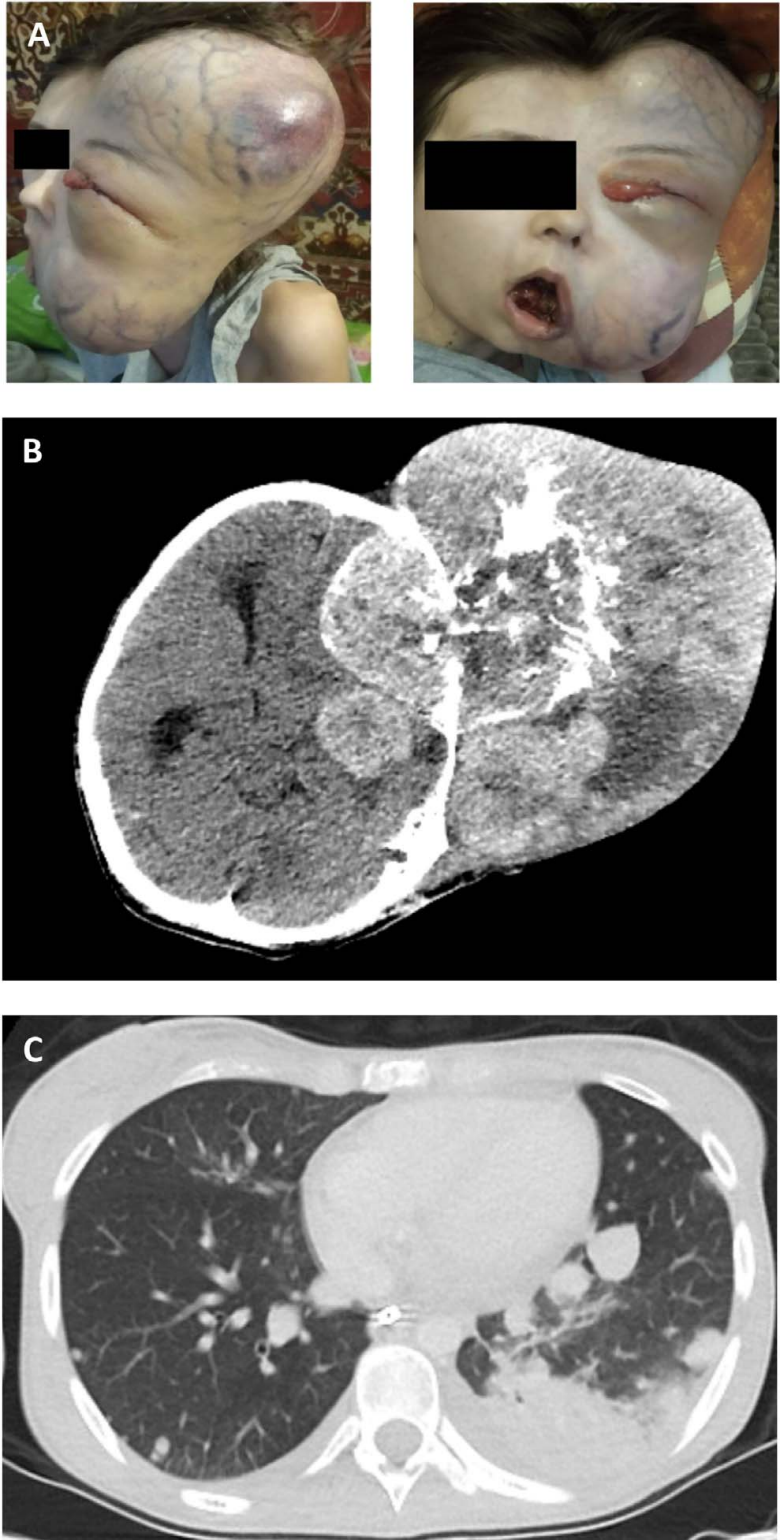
(A) The 20-year-old patient presented with a massive nontender tumor mass on the left temple with visible oral and orbital infiltration. (B) Computed tomography shows a tumor mass of 130 mm × 160 mm × 200 mm in the left hemisphere and (C) pulmonary metastasis.
